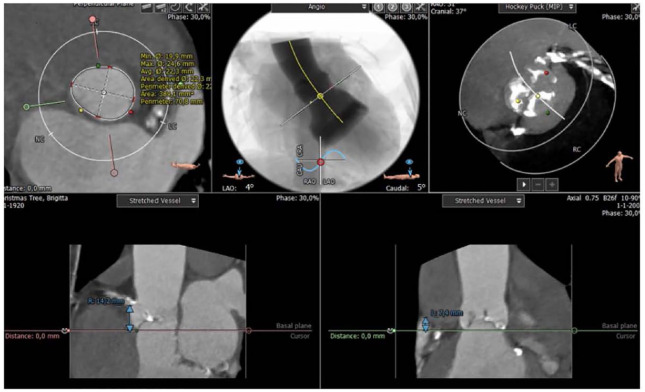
Computed tomography reconstructions for procedural planning with annulus sizing, implant angle, calcium distribution, and distance to coronaries.
